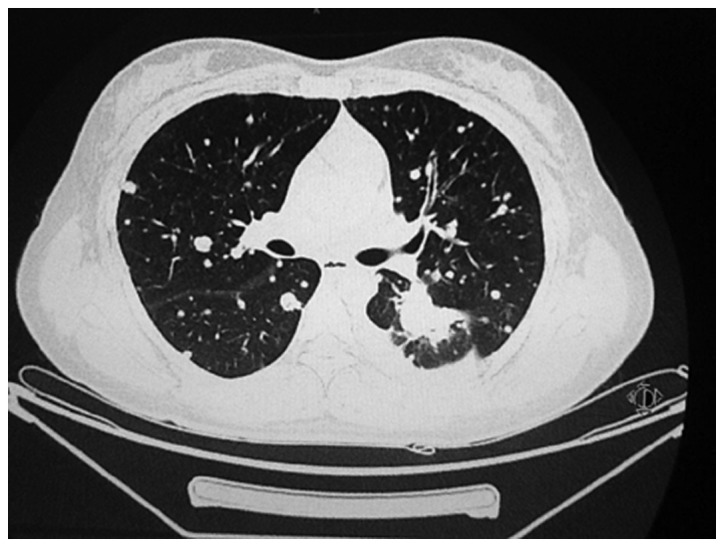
Initial chest computed tomography scan revealing a mass of 31×26 mm in size in the left lower lobe and metastases in each lung.
